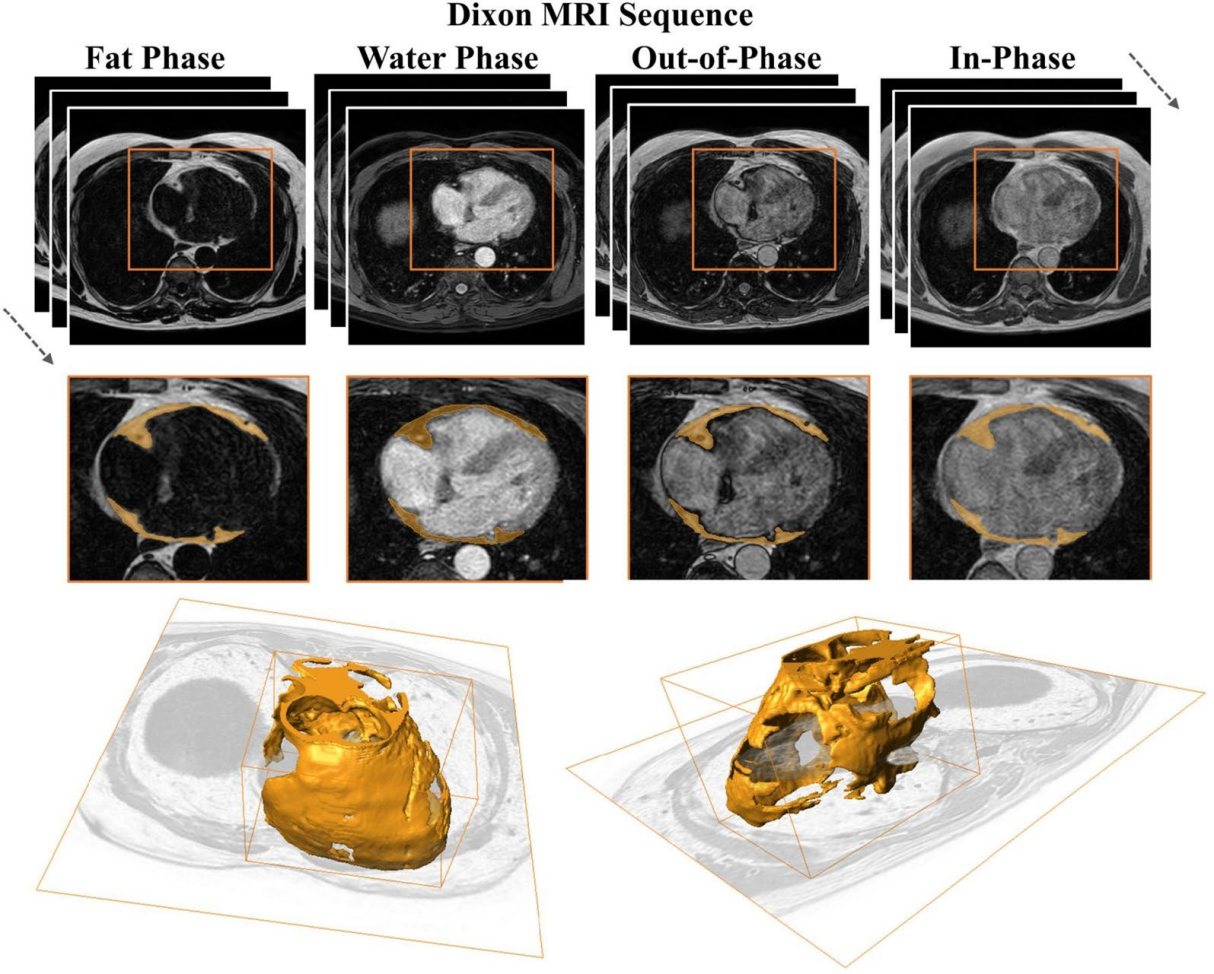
The Dixon MRI sequence consists of four phases: fat phase (FP), water phase (WP), out-of-phase (OP) and in-phase (IP) images. Each phase contains the same number of slices. Epicardial adipose tissue (EAT) is identified as interior pixels highlighted in orange. The different phases show varying contrasts that contain both structural and contextual information. 3D reconstruction of EAT segmentation from Dixon MRI is shown in the bottom row
